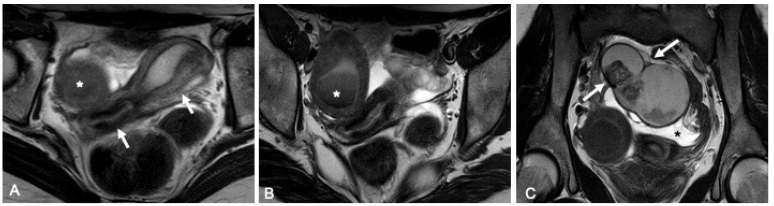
18-year-old teenage girl with pelvic pain. (A) Axial oblique Τ2W MR image of the pelvis shows a left banana-shaped hemiuterus and single cervix (arrows). There is a closely associated right blind rudimentary horn (asterisk). (Β) Axial oblique Τ2W MR image of the pelvis at an upper level demonstrates the right rudimentary horn distended with menstrual fluid (asterisk). (C) Coronal oblique Τ2W MR image of the pelvis in the same patient shows associated right hematosalpinx (arrows) and free pelvic fluid (asterisk). Findings are consistent with left unicornuate uterus with right associated non-communicating uterine remnant with functional endometrium.
